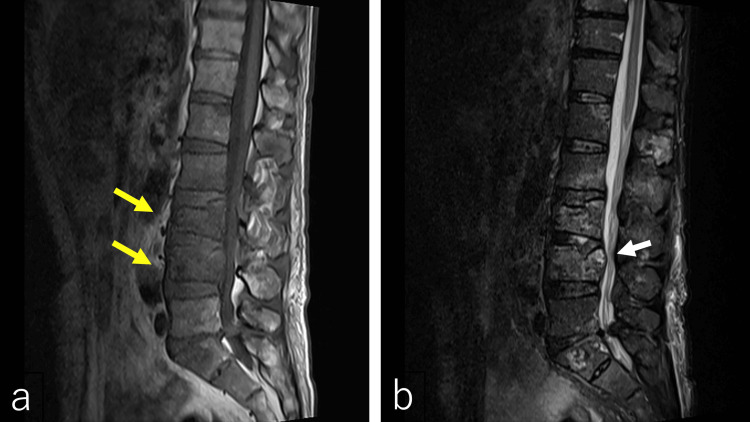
Lumbar spine MRI T1-weighted images showed low-intensity lesions within L2–L4 vertebrae (a, yellow arrows). T2-weighted images demonstrated spinal canal narrowing at the L4 level with anterior displacement of the spinal cord and cauda equina (b, white arrow).
